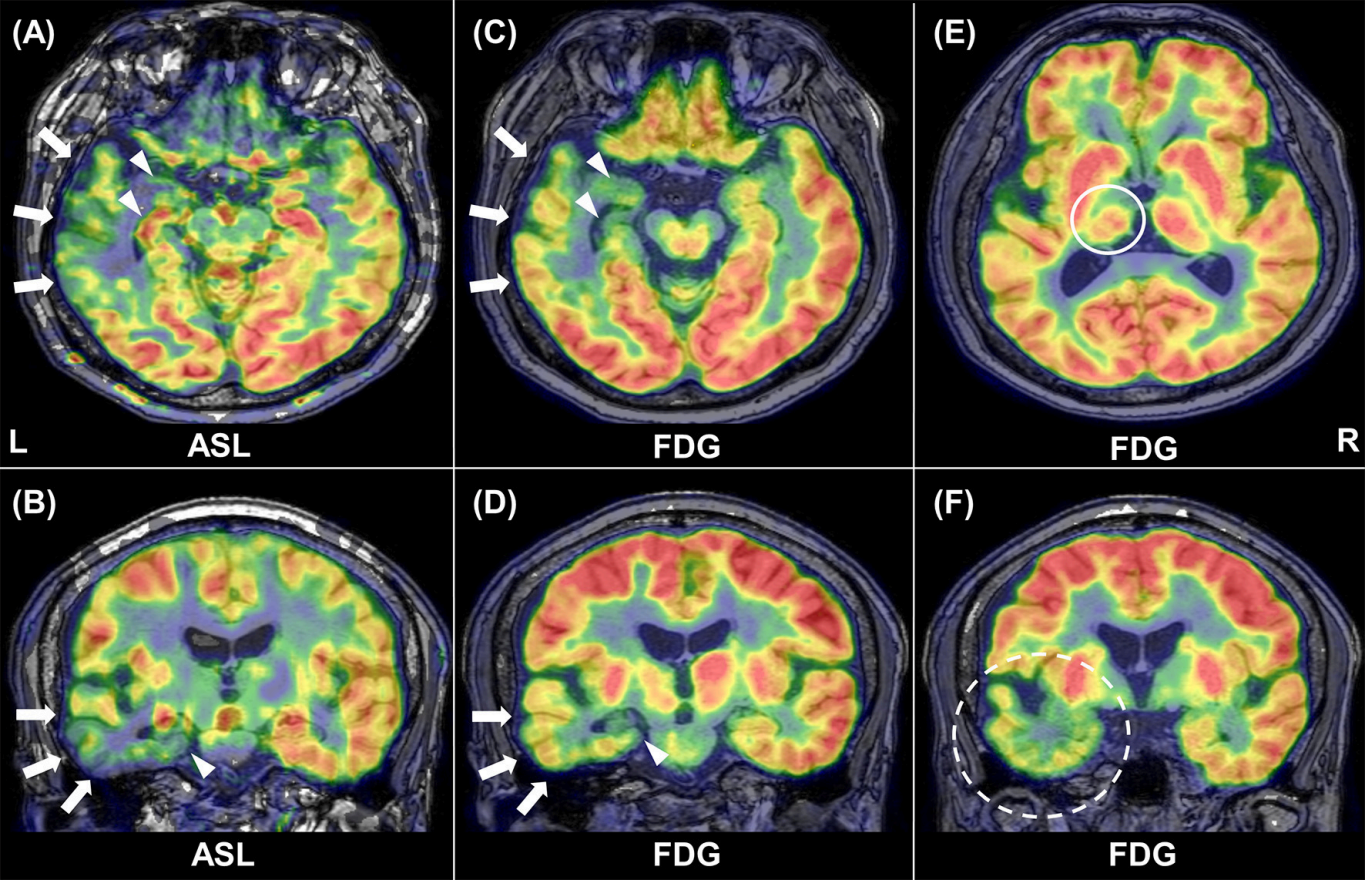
Representative images of ASL and FDG-PET in patient 1. Inter-ictal ASL shows ipsilateral hypoperfusion in the temporal neocortex (arrows) and in the medial temporal lobe (arrowhead) (A, B). Similar findings are present in FDG-PET (C, D). Reduced glucose metabolism can be found in the ipsilateral thalamus [(E), circle] and temporal pole [(F), broken circle].
